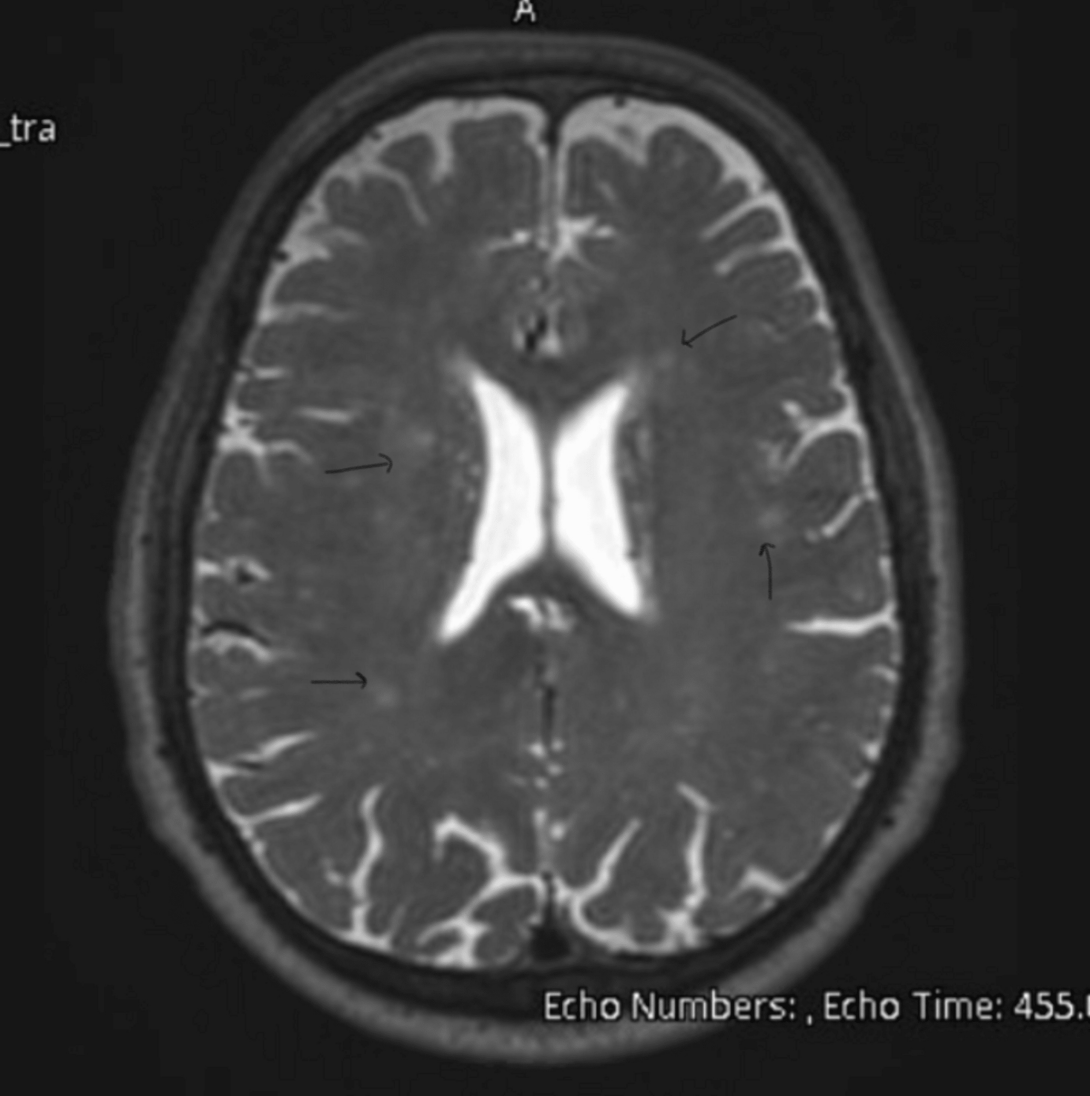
MRI head section 2 showing chronic microangiopathic changes of white matter (black arrows).
